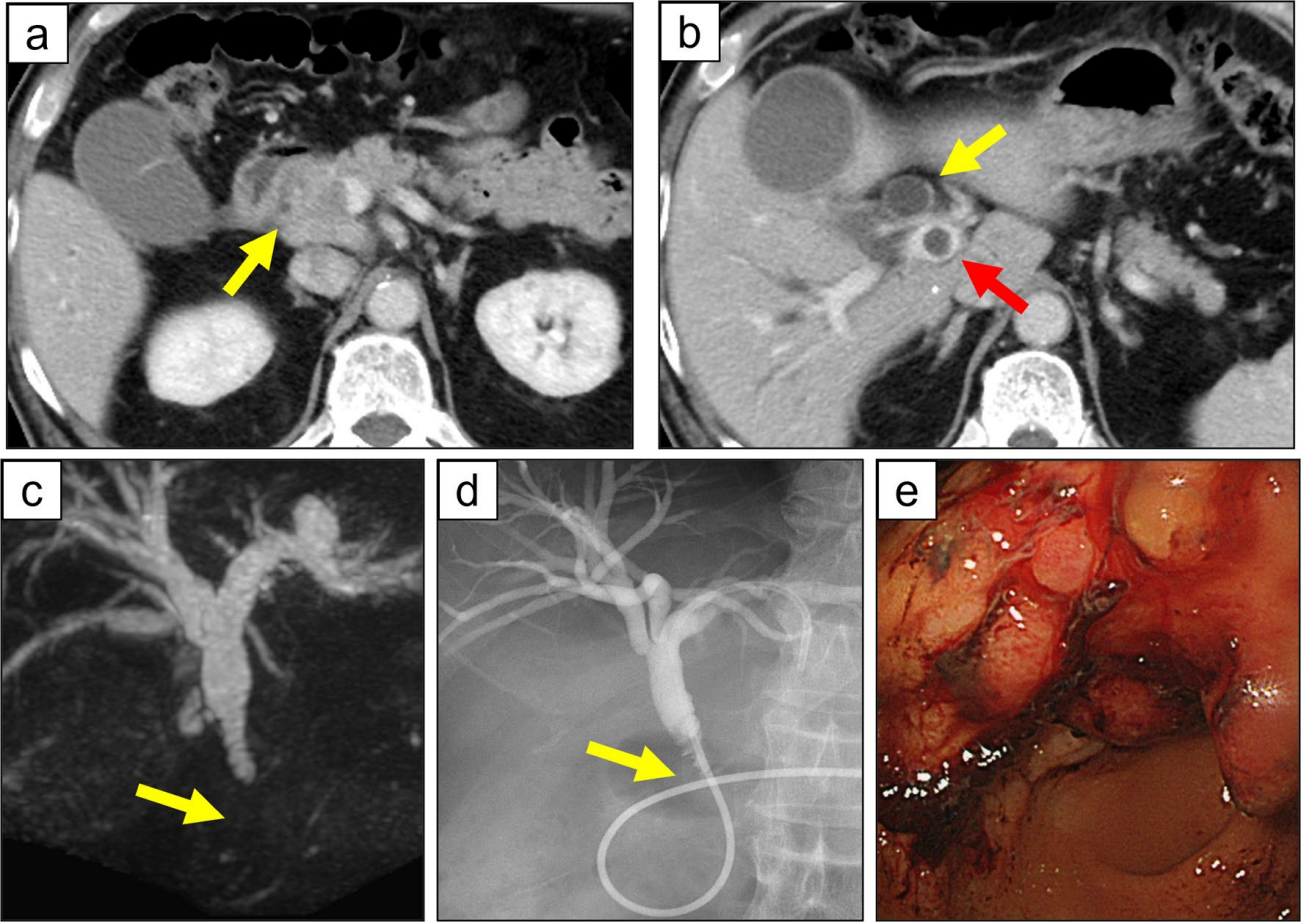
Imaging and endoscopic findings. a Abdominal computed tomography (CT) scan showed wall thickening of the lower bile duct (arrow). b Proximal side of the thickened bile duct was dilated (yellow arrow), and low density area in the portal vein on contrast-enhanced CT scan was considered a tumor thrombus (red arrow). c A defect in the lower bile duct (arrow) and dilatation of the upper side were revealed on magnetic resonance cholangiopancreatography. d Cholangiography showed the same findings as magnetic resonance cholangiopancreatography. The arrow indicated a defect in the lower bile duct. e Gastroduodenoscopy showed a type 3 tumor at the lesser curvature of the gastric antrum
